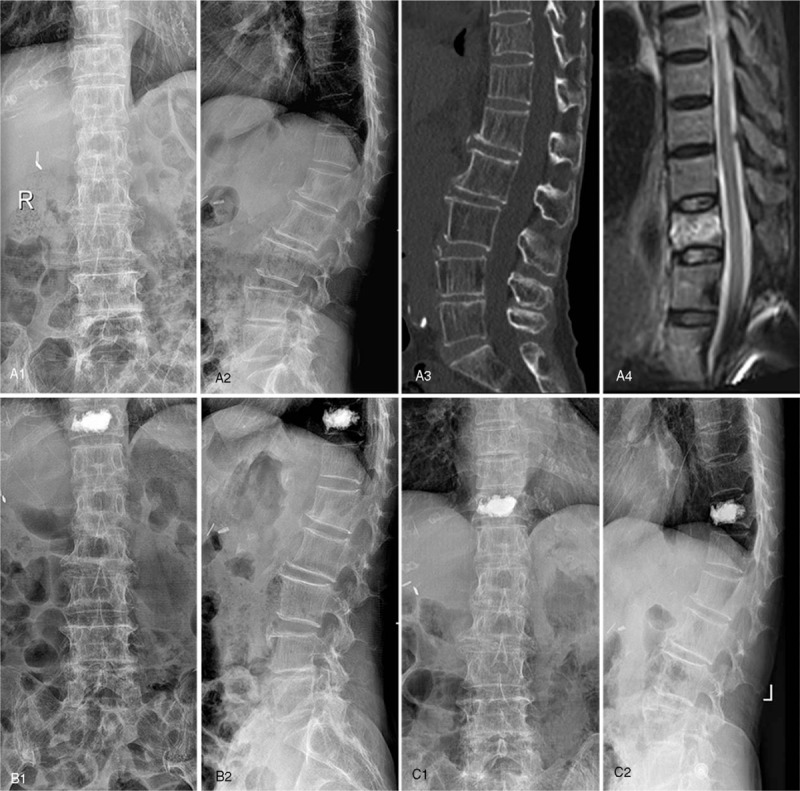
A 77-year-old female diagnosed as T11 OVCF with “O” shaped cement filling pattern. Preoperative X-ray from coronal plane (a1) and sagittal plane (a2), CT image (a3), and MRI fat suppression image (a4). Postoperative X-ray from coronal plane (b1) and sagittal plane (b2) at 2-days follow-up. Postoperative X-ray from coronal plane (c1) and sagittal plane (c2) at 1-year follow-up.
